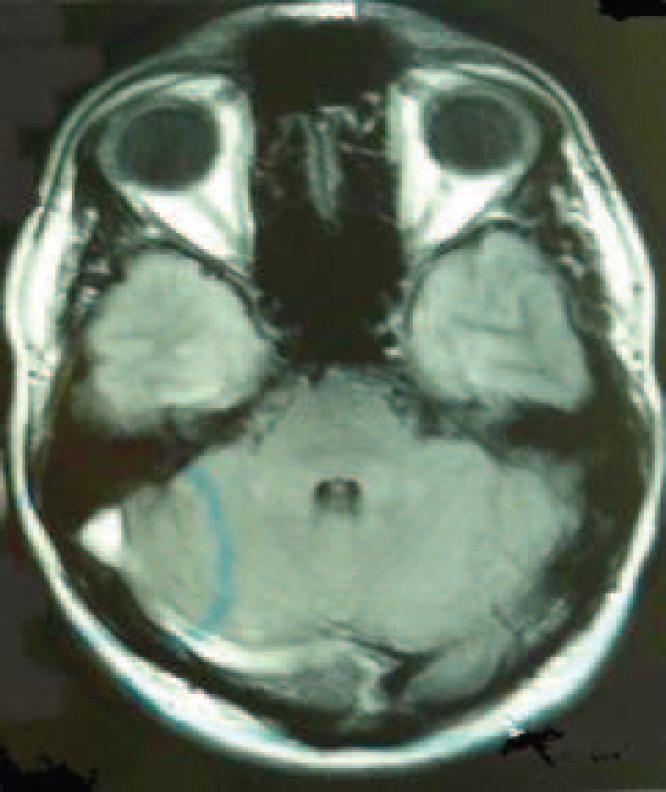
Axial brain MRI in FLAIR sequence showing abnormal signals in sigmoid sinus.
